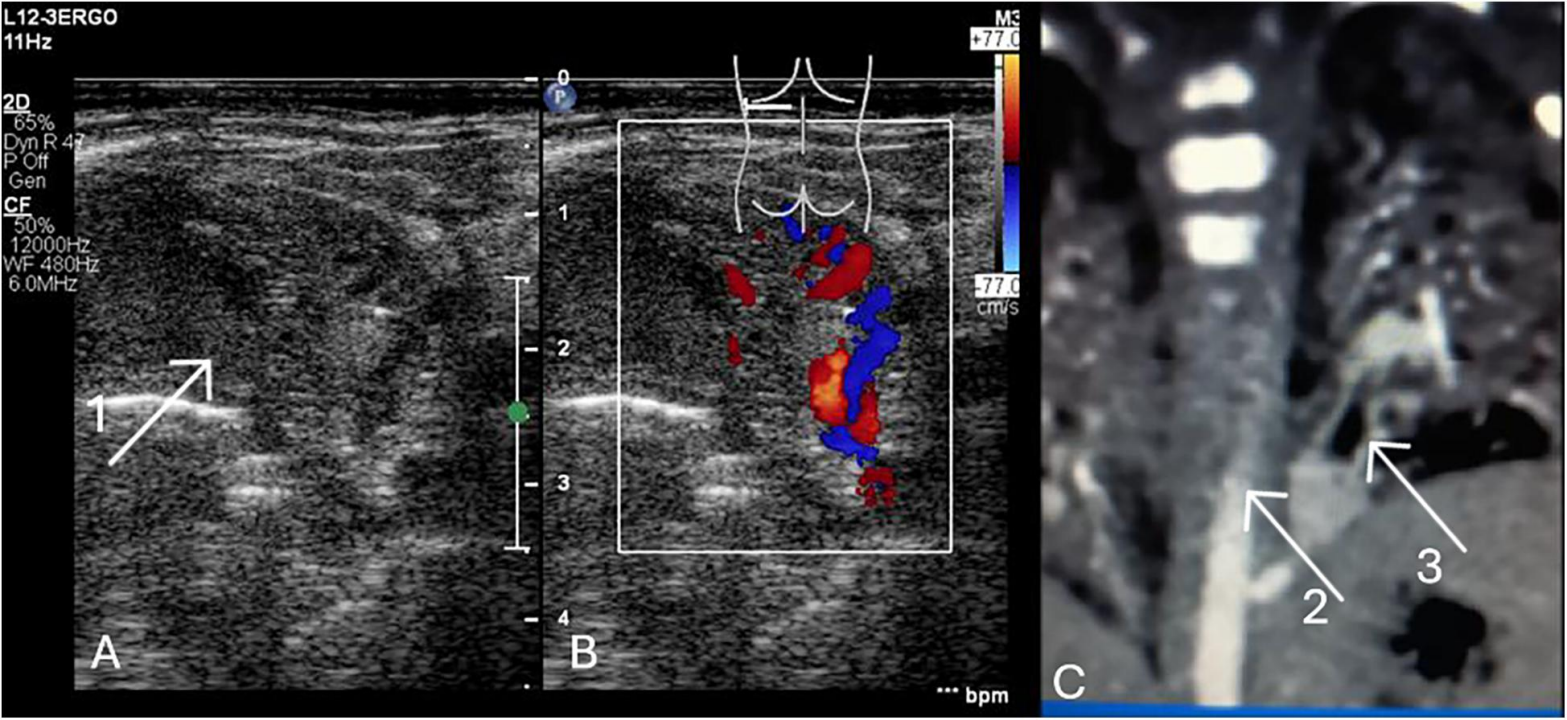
(A) LUS. Intrapulmonary sequestration—atelectatic area with heterogeneous structure, mostly solid (1). Transversal position of the linear probe, supradiaphragmatic region of the posterior field of the left lung. (B) LUS. The vessels marked with color Doppler are branches of the aorta. (C) Angio CT. The lesion (2). Aortic branch (3). The male neonate was admitted with respiratory failure (nCPAP, FIO_2_ 0.4, RDS) following caesarean section at 37 weeks gestation, with a birth weight of 3,320 g and an Apgar score of 9. The mother received lamotrigine and lacosamide for epilepsy during pregnancy. Prenatal imaging indicated possible pulmonary sequestration. Echocardiography revealed an atrial septal defect and a right subclavian artery arising from the aortic arch. The respiratory disorder resolved, and the patient was discharged with a plan for outpatient follow-up at a surgical clinic in 2–3 months to assess and schedule resection of the pulmonary sequestration.
